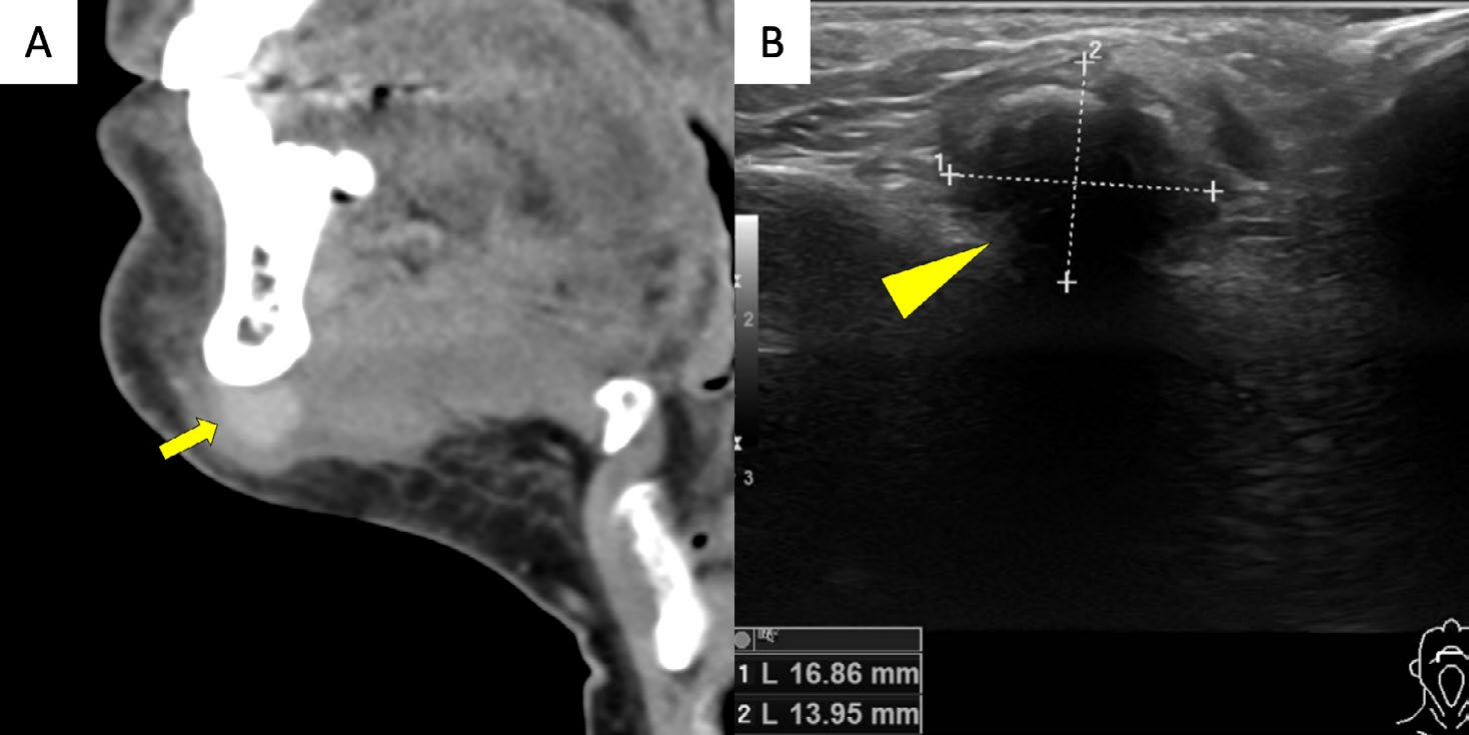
Computed tomography imaging and ultrasonographic imaging. (A) Sagittal view shows a contrast‐enhancing mass (open arrow) abutting the mandible with suspected infiltration of the digastric muscle and thickening of the adjacent platysma, but without evidence of sclerotic changes. (B) In the ultrasound, a submental mass (arrowhead) with an irregular hyperechogenic border is seen within the digastric muscle, accompanied by a posterior acoustic shadow.
